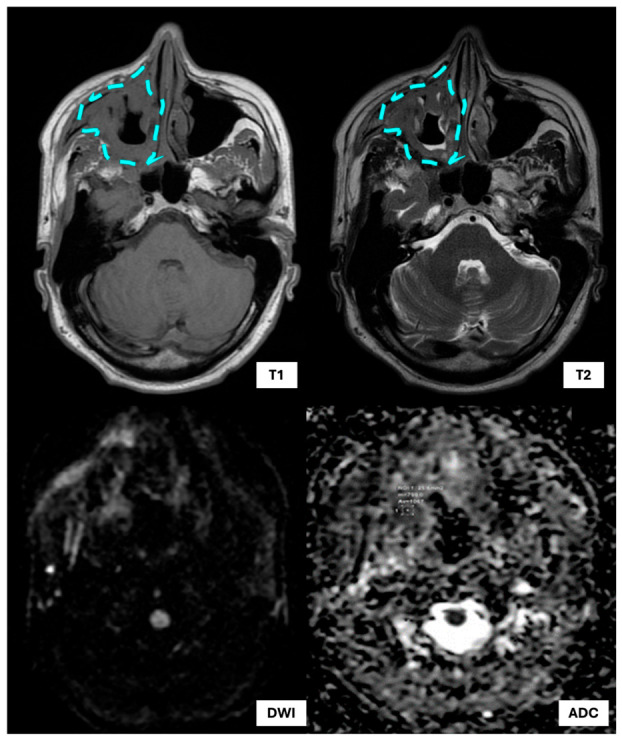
A 39-year-old patient presenting with right cheek anesthesia. MRI demonstrates a circumferential soft tissue mass with low signal intensity on T1- and T2-weighted images, involving the right maxillary sinus (blue dashed line) and extending into adjacent structures, including the ethmoidal cells, nasal cavity, pterygopalatine fossa, skull base, and sphenoidal sinus. DWI and the corresponding ADC map show restricted diffusion (ADC value = 1.06 × 10^−3^ mm^2^/s). The final diagnosis was adenoid cystic carcinoma.
